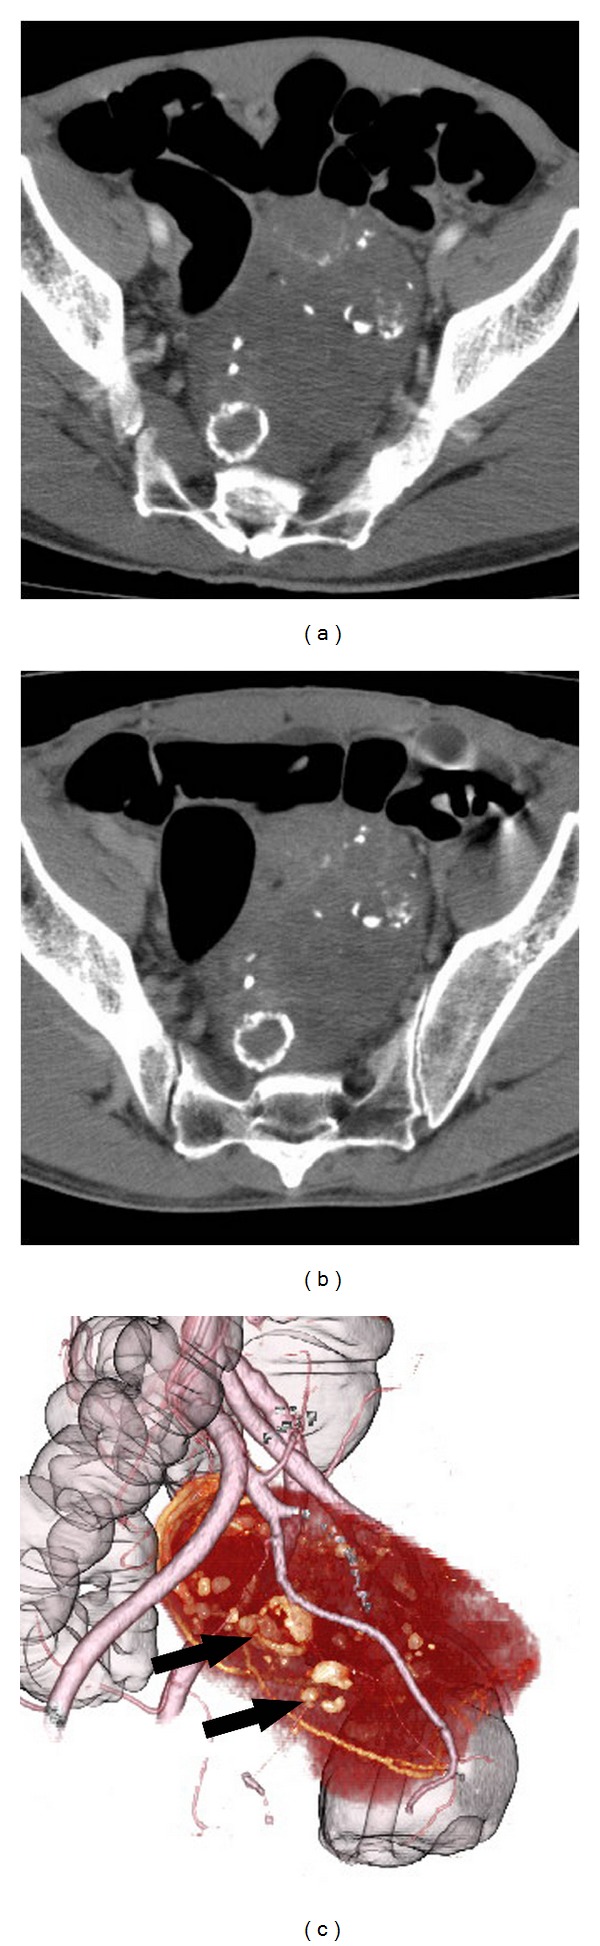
(a) Contrast-enhanced CT shows a low-density mass containing coarse and round calcifications in early phase (40 s). Cystic lesions are not evident. (b) The mass is slightly enhanced in delayed phase (180 s). (c) 3D-CT shows eggshell-like calcifications within the tumor (arrows).
